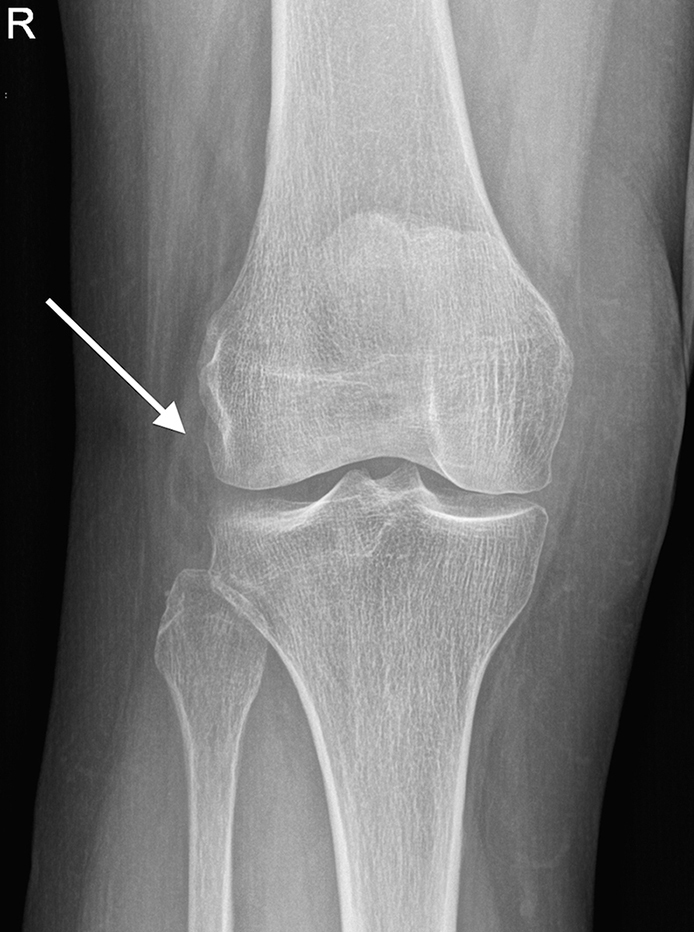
Anteroposterior radiograph of the right knee shows a subtle low-density structure adjacent to the lateral joint space, suggestive of a fat-containing lesion (arrow).
